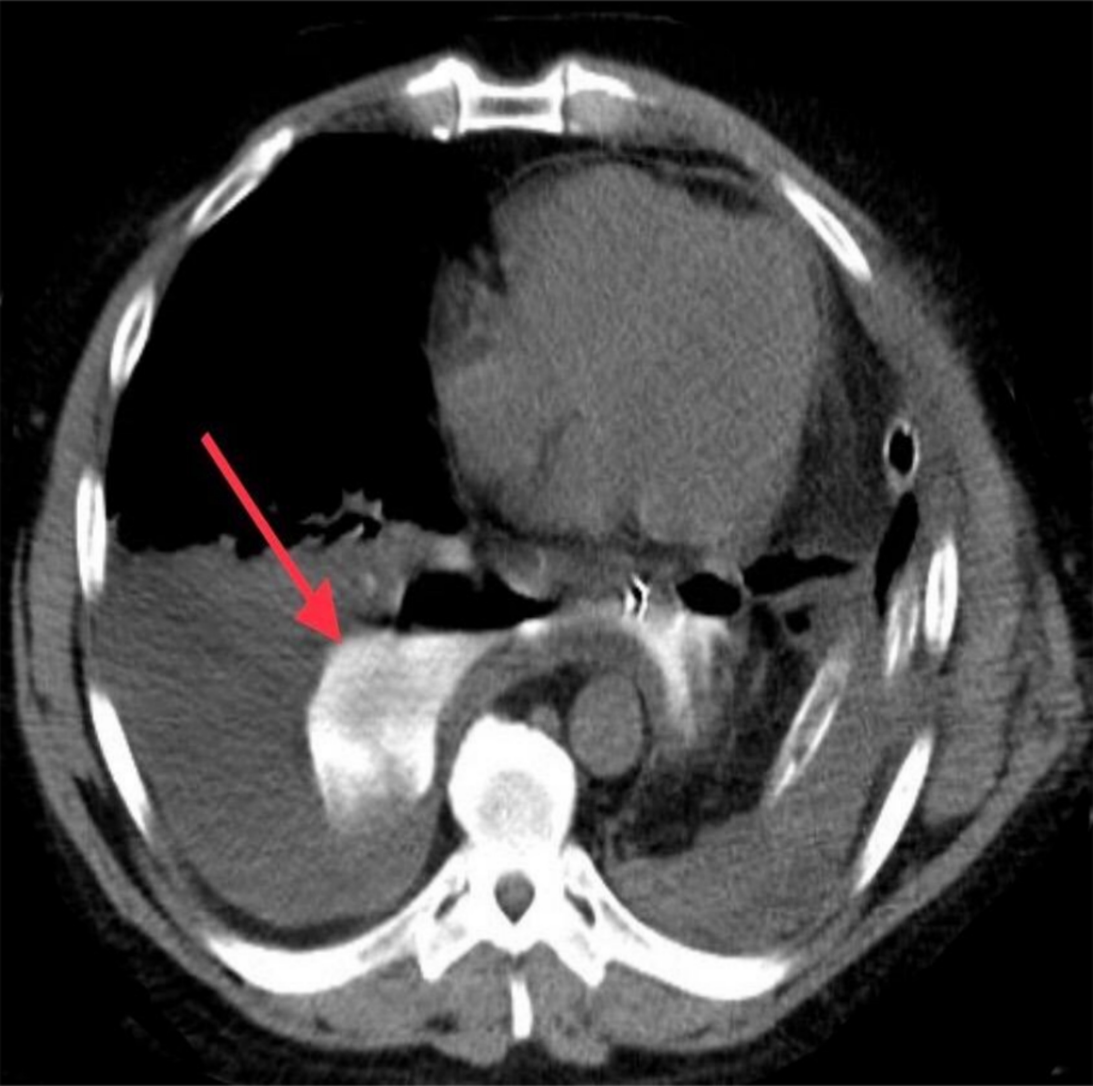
Chest CT scan with oral contrast Anastomotic leak into the right pleural cavity (red arrow)
